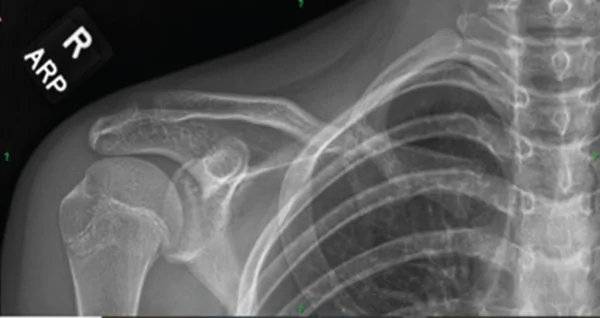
Day 31 radiographs demonstrating progressive callus maturation at the medial clavicle fracture site.
